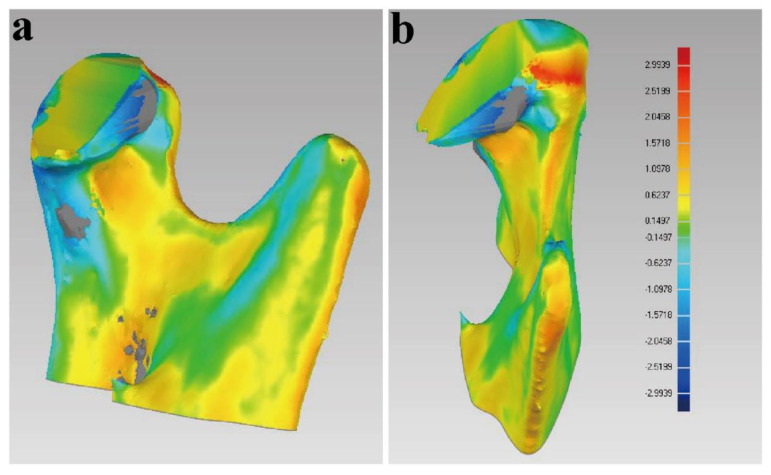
Evaluation of the accuracy of DOS and DOT: (a,b) superimposition of the virtual condylar segment and the postoperative CT scan at 1 week after surgery, for accuracy analysis.
